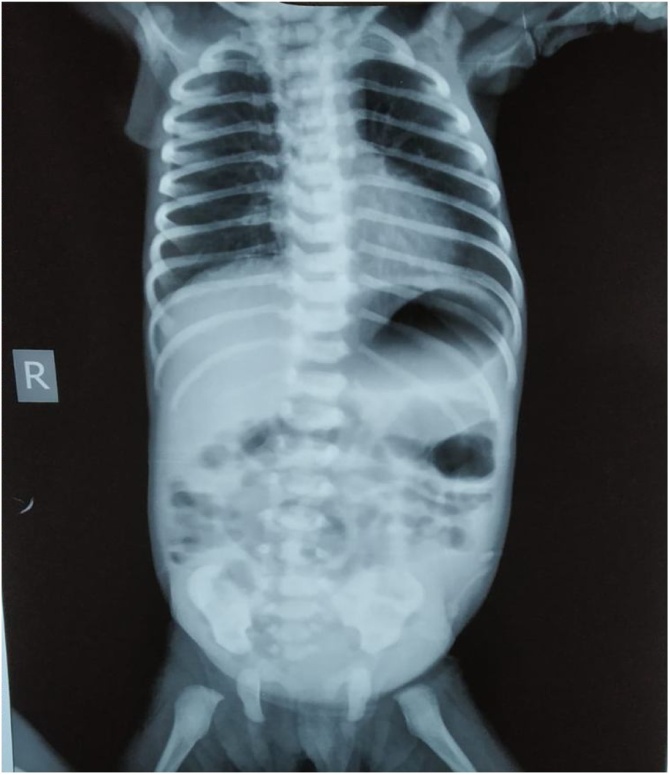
X-ray plain picture abdomen of the infant.
